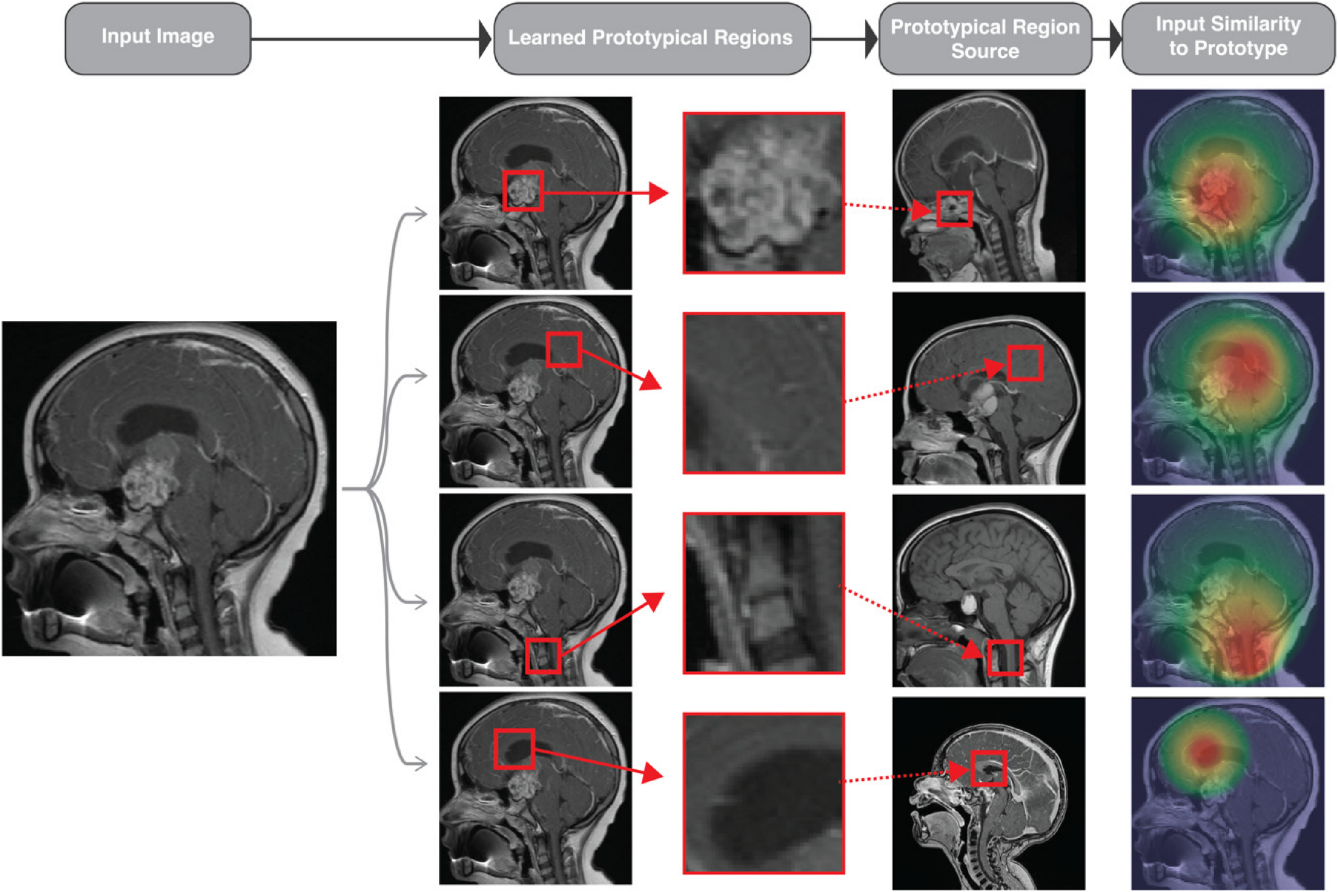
Conceptual rendering of how the “This Looks Like That” (protoPNet) approach could be applied to classify a single sagittal MR image. The input image (left) is a preoperative sagittal contrast-enhanced T1-weighted MR image of a patient diagnosed with a suprasellar tumor.67 The second column shows overlaid bounding boxes generated by a model, highlighting the content like the prototypical parts learned by the algorithm. The third column displays the prototypical parts learned by the model, while the fourth column presents the source images of the prototypical parts. Finally, the rightmost column shows the activation maps indicating the extent to which each prototypical part resembles the test image, with red indicating high and green indicating low resemblance.
